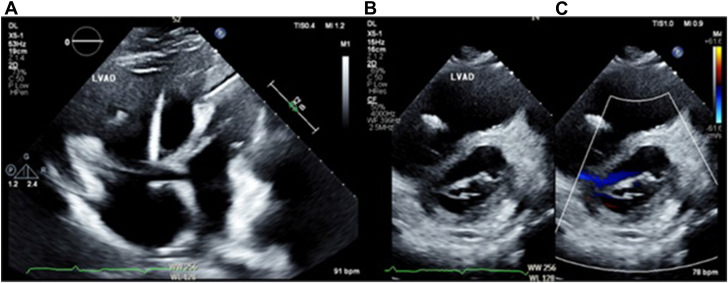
Pre-Impella implantation transthoracic echocardiogram (TTE) findings. (A) Apical 4-chamber TTE view. Massive RV dilatation concerning for acute RV failure can be seen. (B and C) Parasternal short-axis TTE view. A D-sign, pressure, and volume overload with an underfilled LV can be discerned.
